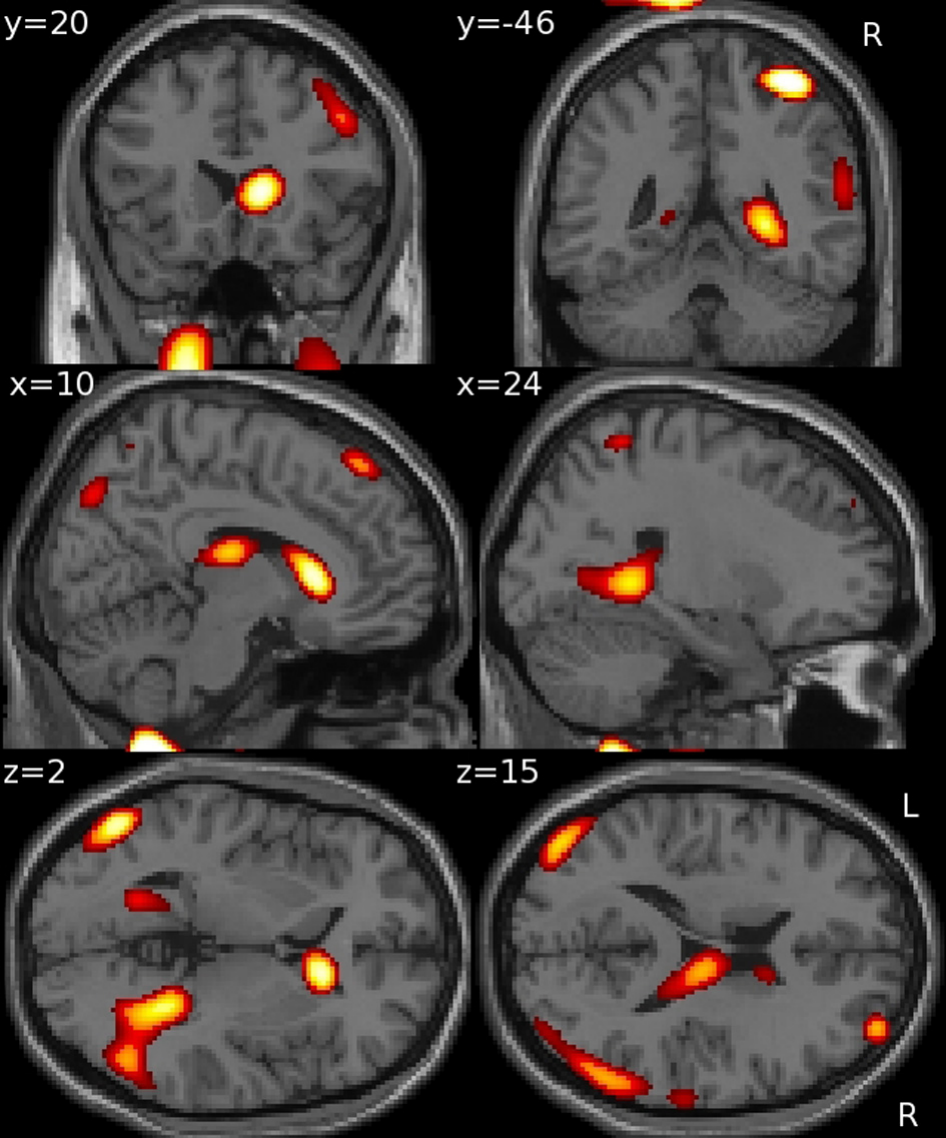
Multivariate support vector regression of VRLT performance with normalized FDG metabolism.
